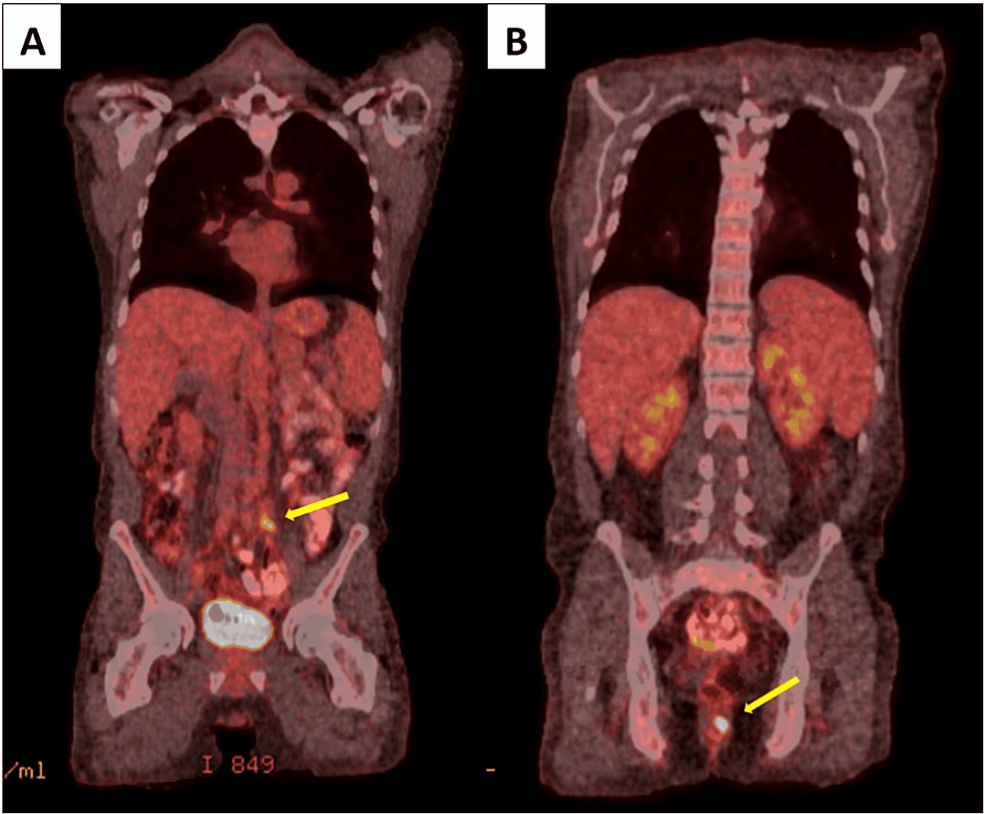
(A) Focus of high FDG avidity adjacent to the left common iliac artery, suspicious for metastatic disease (arrow). (B) Focus of high FDG avidity within the rectum, suspicious for malignancy (arrow).
